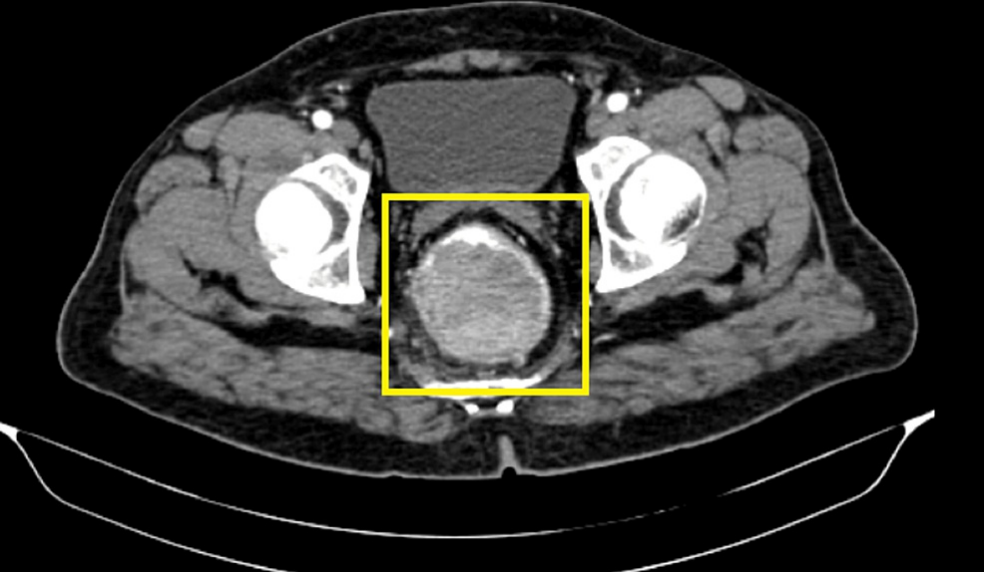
CT image showing a polypoidal lesion
